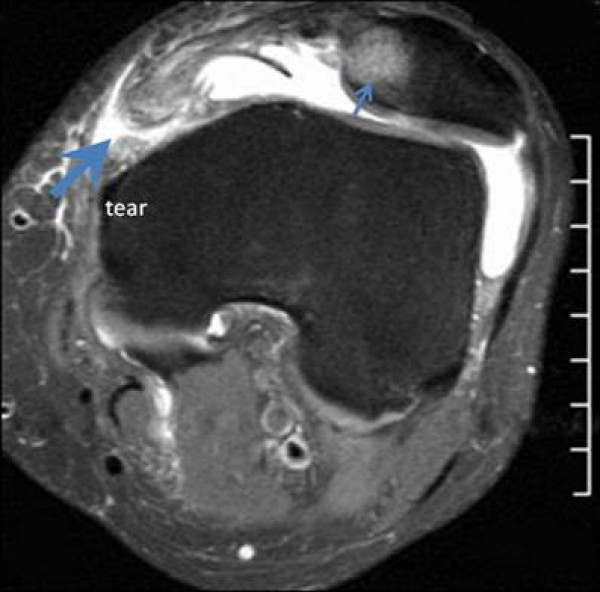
Axial T2 MRI image indicates tearing at the femoral attachment (large arrow) and bone contrusion at the medial patellar facet (small arrow).
